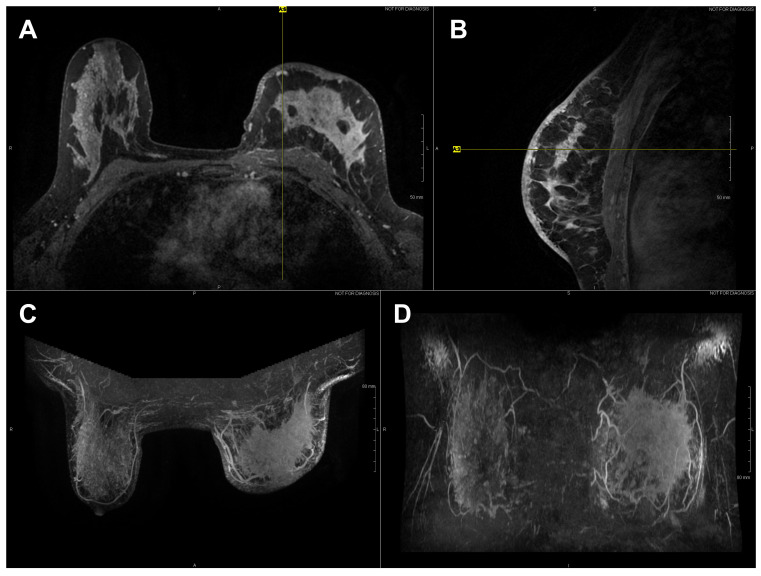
Axial (A) and sagittal (B) T1 contrast enhanced MR images of Subject 03’s malignant lesion. Axial (C) and coronal (D) maximum intensity projection images demonstrating large left breast mass and overlaying vasculature. Linear enhancing vessels on sagittal images appear as enhancing round or oval foci on axial cross-sections.
